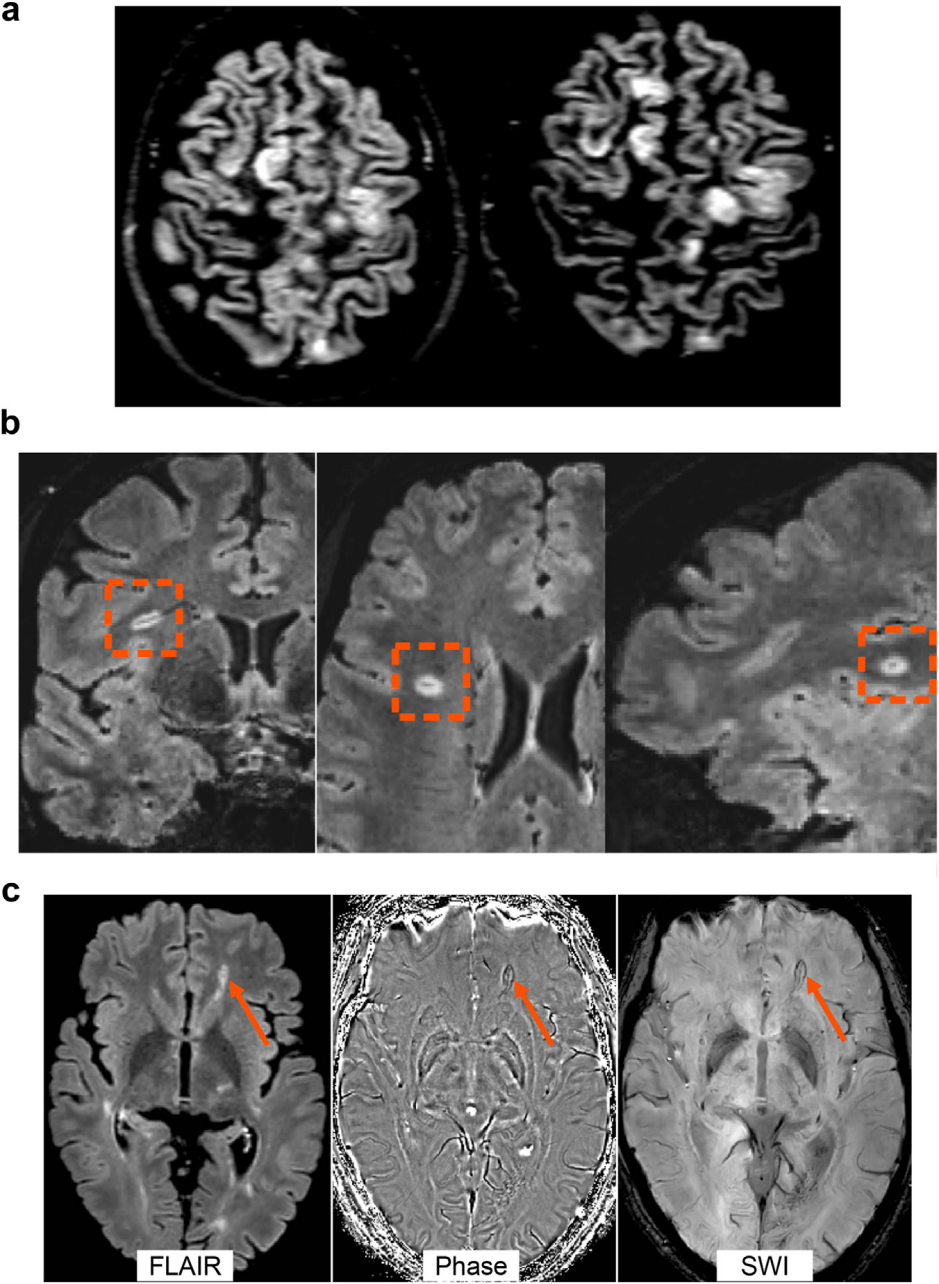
Examples of cortical lesions, the central vein sign and chronic active lesions. (a) Several cortical lesions are visible in a multiple sclerosis patient on double inversion recovery sequence at 3.0 T. (b) A white matter lesion showing the central vein sign (orange dotted rectangle) on post-contrast T_2_-FLAIR∗ sequence at 3.0 T. (c) Among the T_2_-hyperintense white matter lesion visible on T_2_-FLAIR, one lesion shows an hypointense rim on phase image and SWI sequence at 3.0 T (orange arrow). Abbreviations: FLAIR, fluid-attenuated inversion recovery; SWI, susceptibility-weighted imaging.
